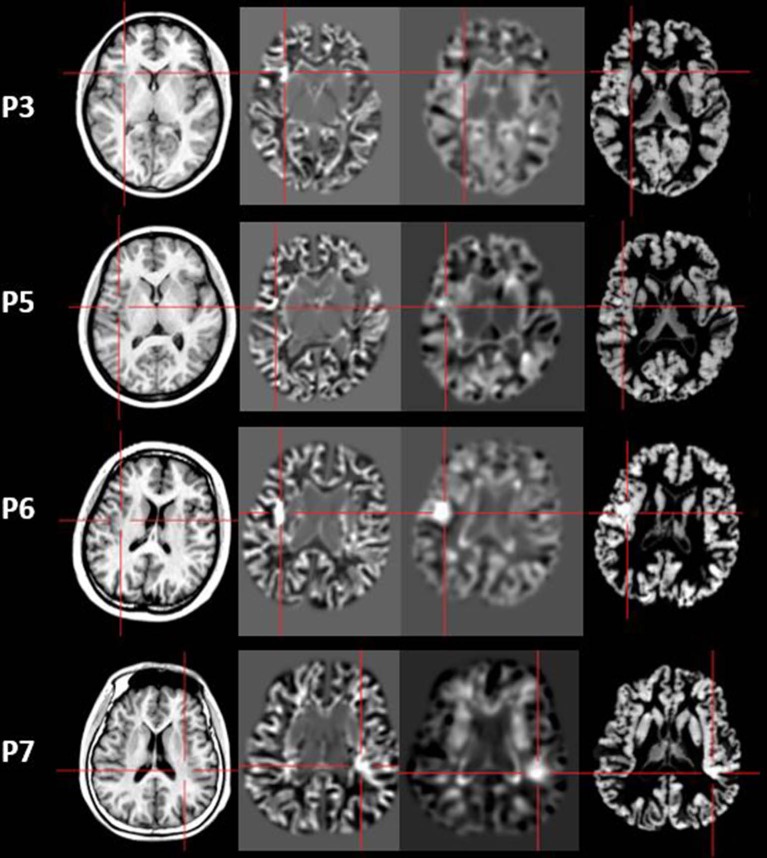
The junction z-score images, extension z-score images, and thickness z-score images of MAP-positive patients (Patient 3, 5, 6, and 7). First column: T1-weighted magnetization prepared rapid acquisition with gradient echo (MPRAGE) images used as input to MAP. Second column: junction z-score image of MAP. Third column: extension z-score image of MAP. Fourth column: thickness z-score image of MAP. All of the four patients had positive results on junction z-score images. However, only two patients (P6 and P7) showed positive results on extension z-score images and thickness z-score images.
